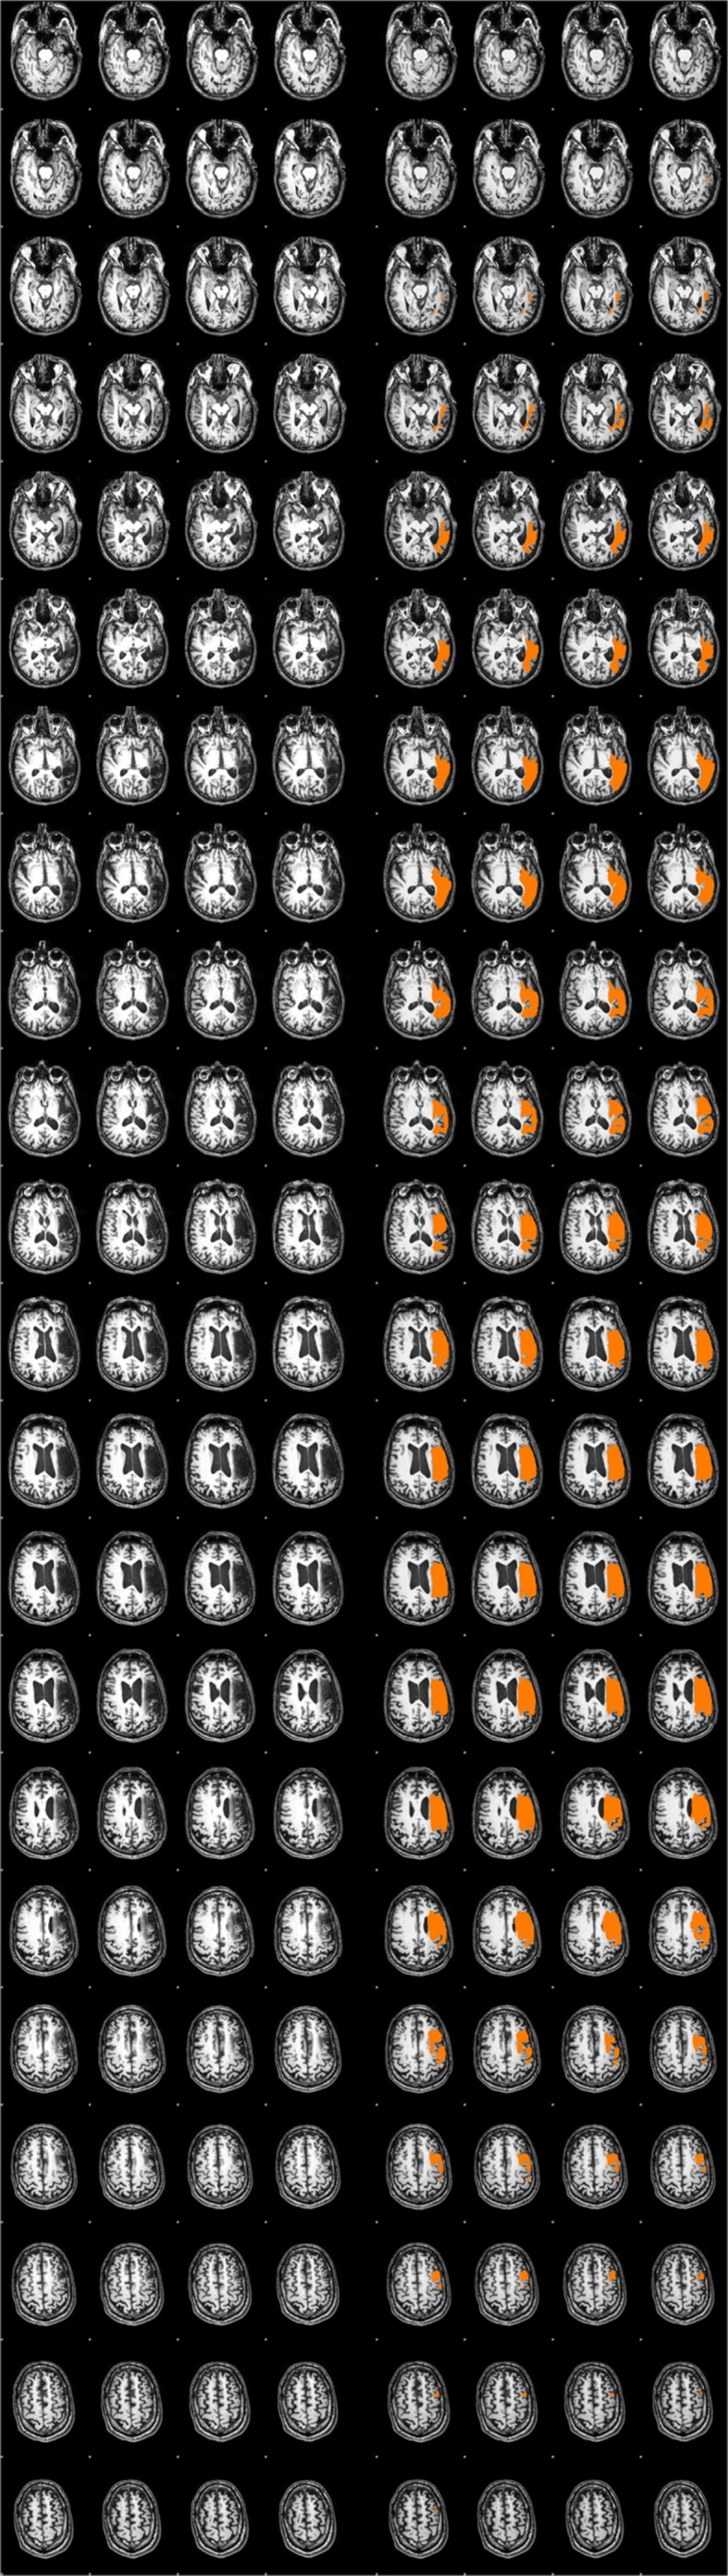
Side-by-side comparison of Participant 8’s T1-weighted MRI scan, with and without the lesion mask, revealing viable tissue in the both ROIs.
